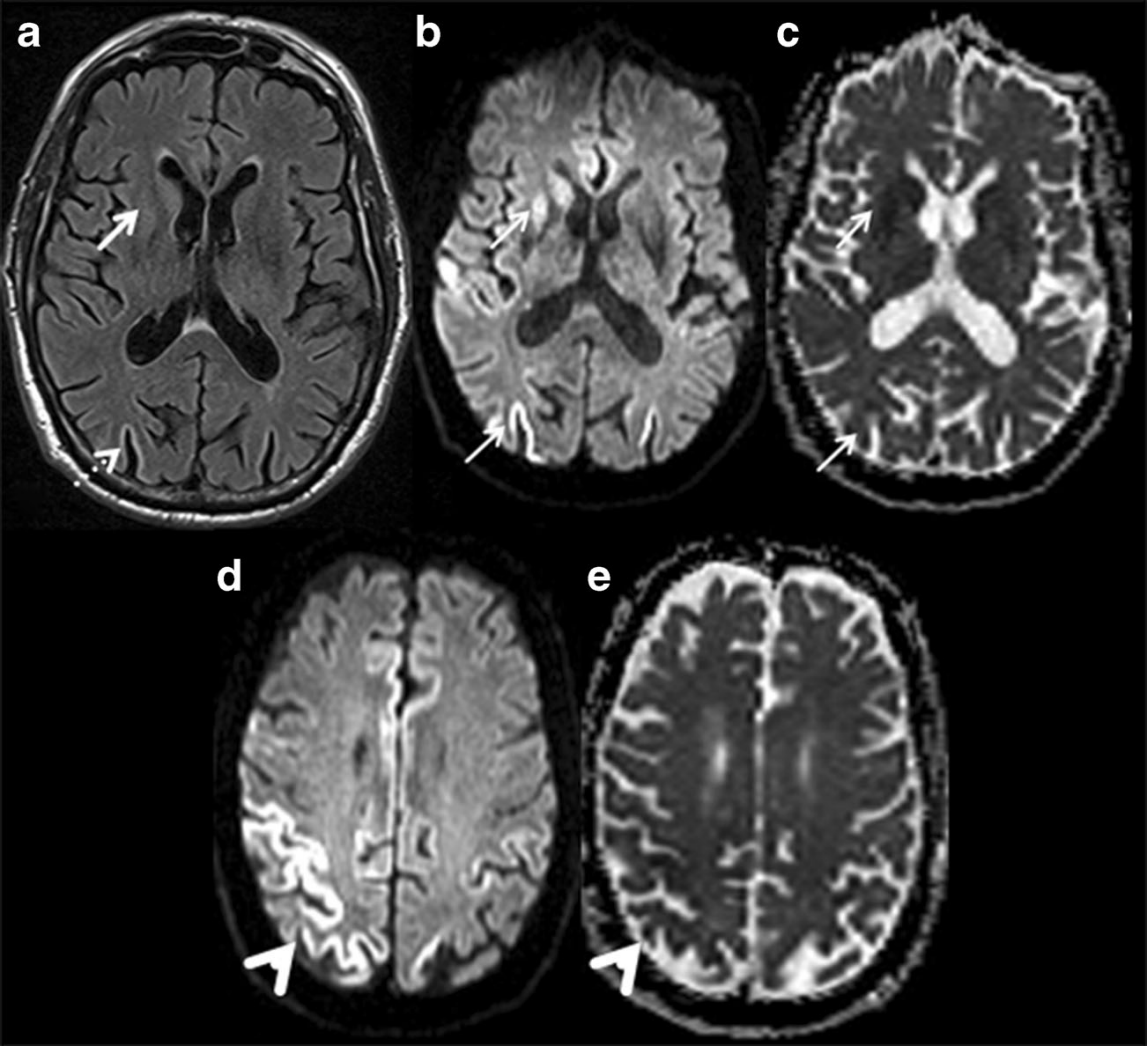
Creutzfeldt-Jakob Disease (CJD). a FLAIR axial image of the brain shows hyperintensity in the right caudate head, putamen (*white arrow*) and occipital cortex (*white dashed arrow*). b & c diffusion weighted image and ADC map reveal restriction of diffusion in the right caudate head, putamen and occipital cortex. d & e diffusion weighted image and ADC map at higher level demonstrate restriction of diffusion in the right frontoparietal cortex
